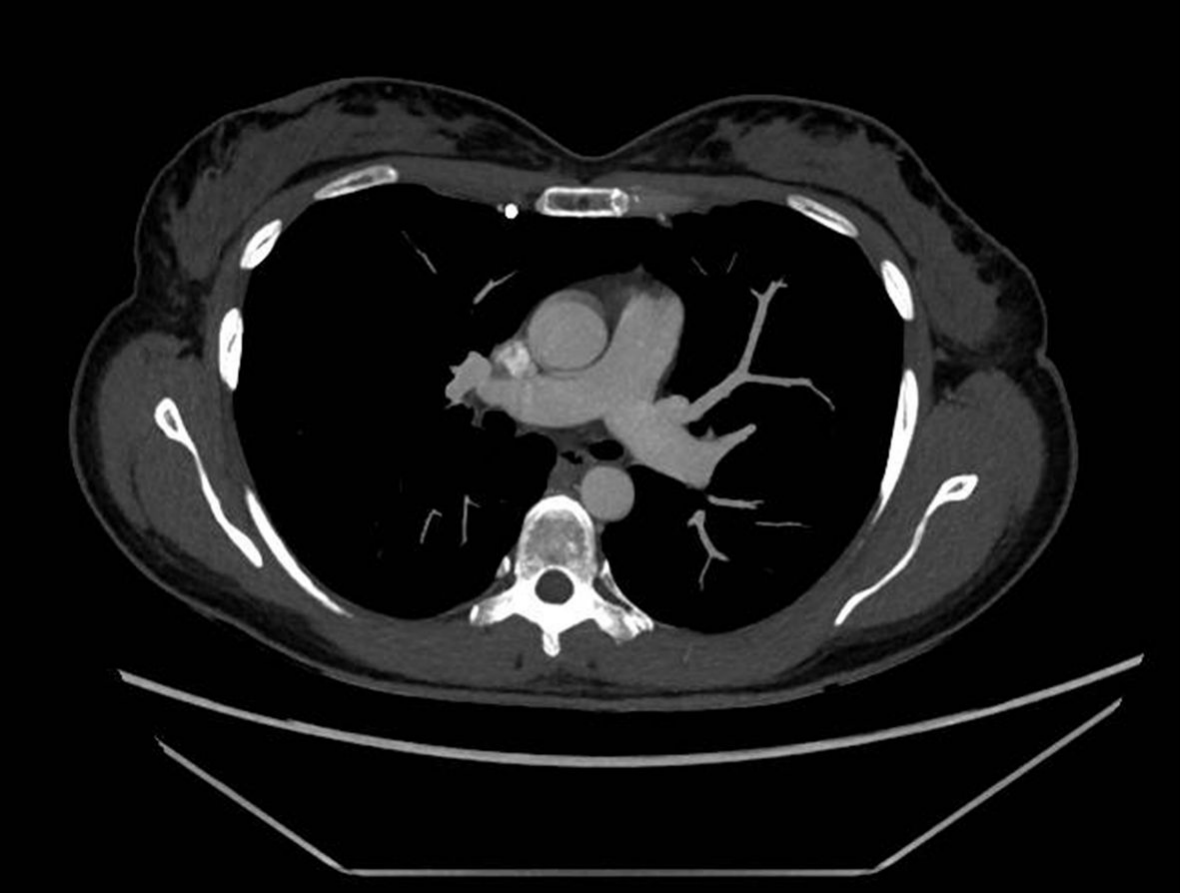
Axial computed tomography image showing the port-a-cath positioned behind the anterior chest wall.
